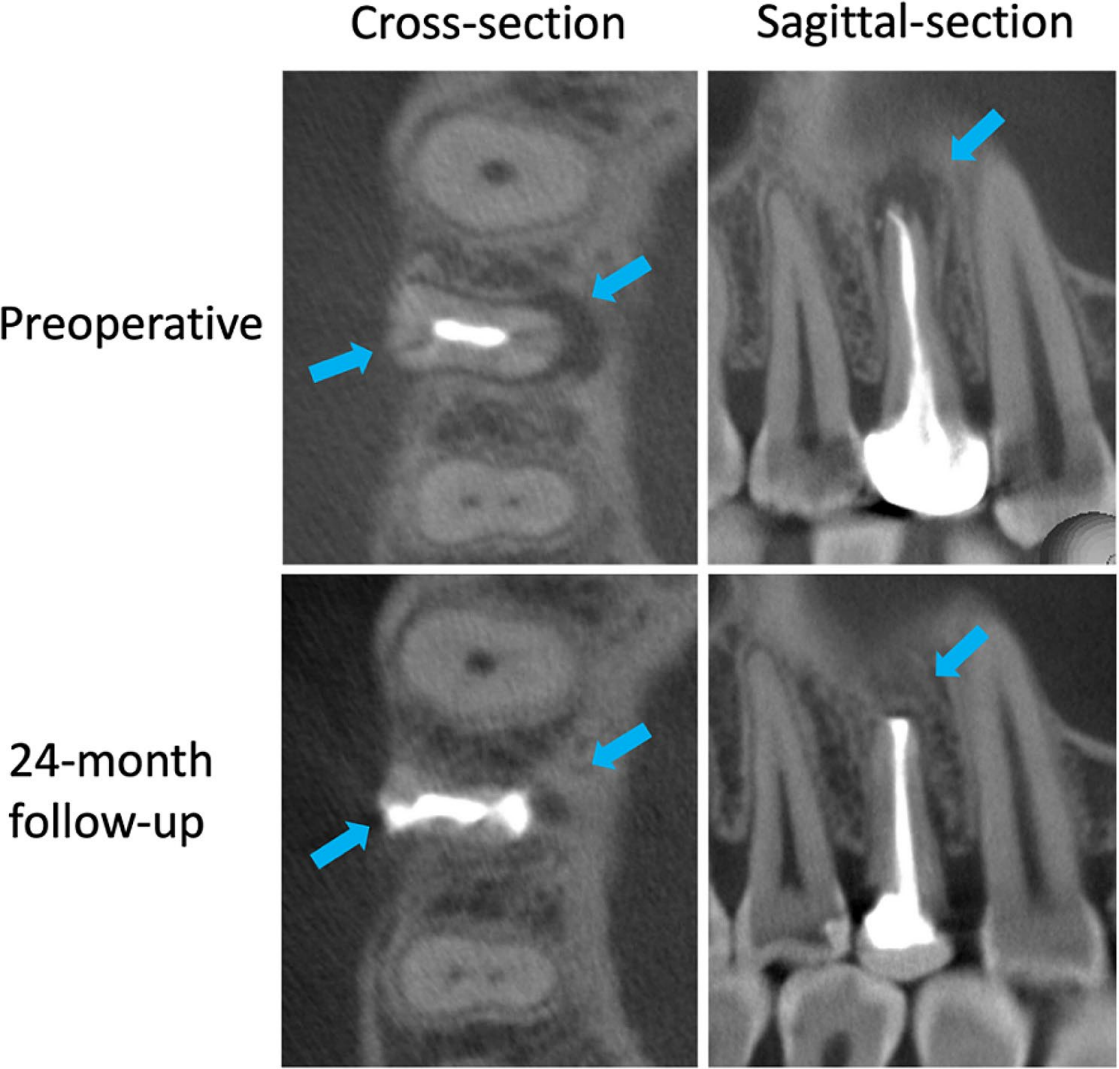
CBCT cross and sagittal images of tooth #14 with VRF before surgery and at 24-month review (arrows showing the root fractures, periodontal defect status or filling materials)
